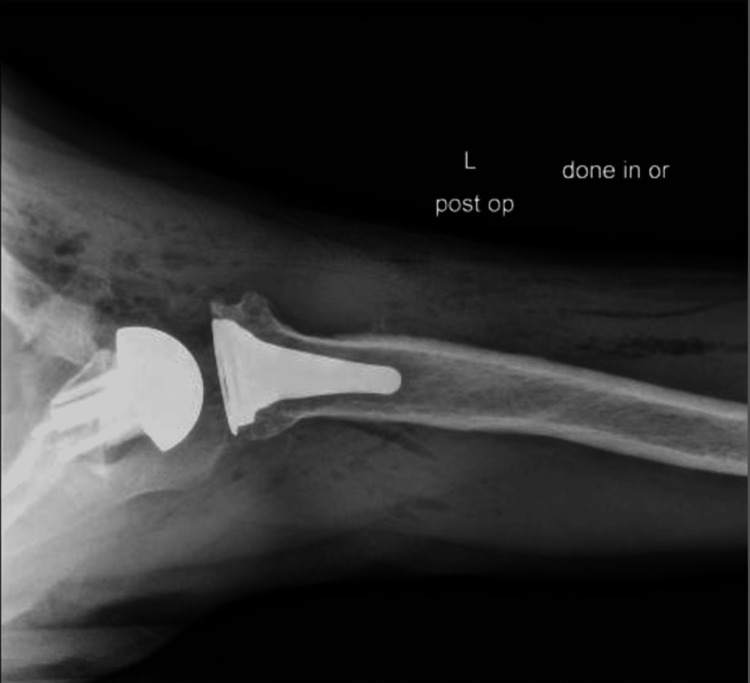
Postoperative X-rays of the left shoulder following rTSA with custom glenoid implant rTSA: reverse total shoulder arthroplasty The image depicts an axillary view of the right shoulder
